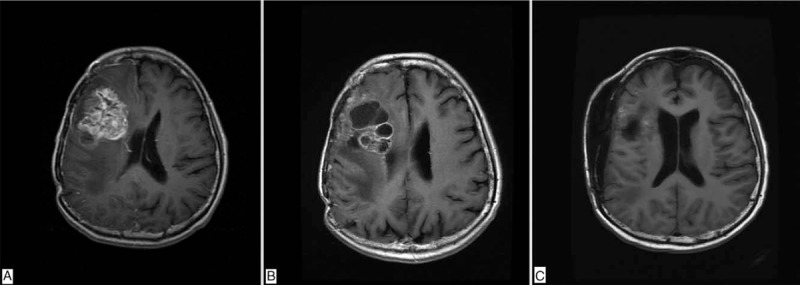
Serial images of brain MRI. (A) Tumor recurrence 20 years after first surgery and RT. (B) Fourth tumor recurrence 25 years after initial diagnosis. (C) Latest MRI of the brain showed that the disease is under control after fourth surgery.
